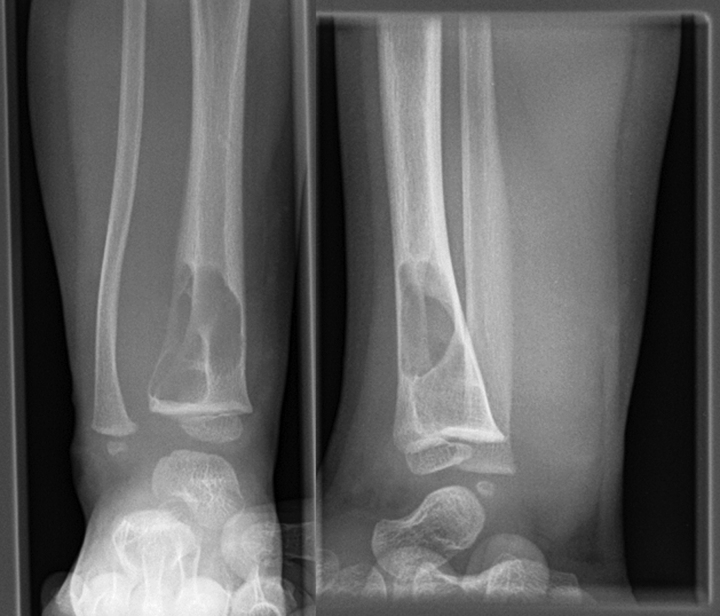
Plain radiographs show an osteolytic lesion of the distal tibial metaphysis.
